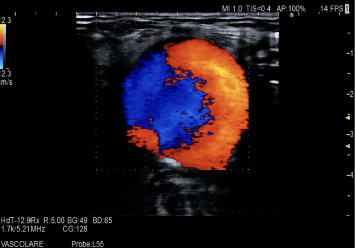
Elliptical pseudoaneurysm arising from a collateral branch, grayscale ultrasound.
